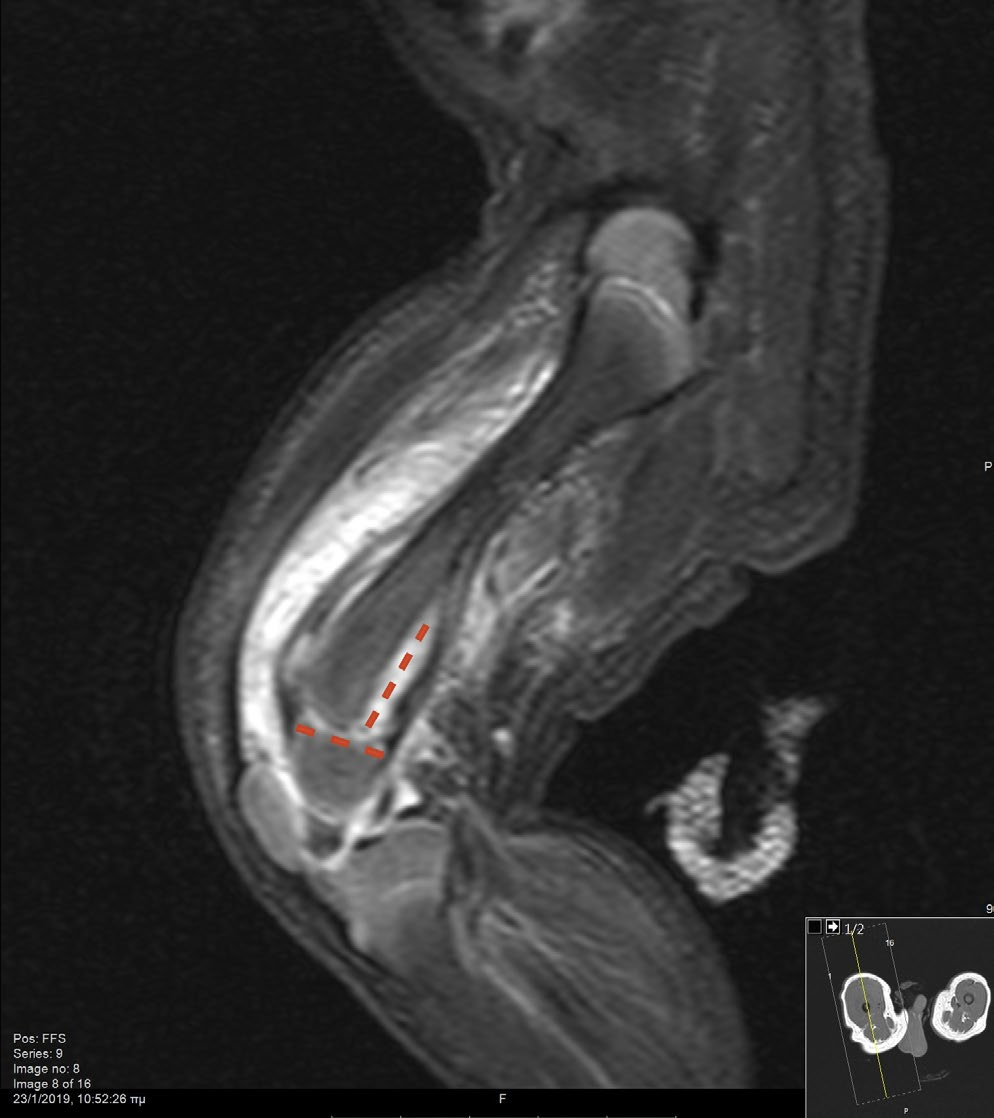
MRI of the right femur depicting the presence of hematoma and disruption of periosteum, setting the diagnosis of Salter‐Harris type II fracture. The red lines indicate the type of the fracture
